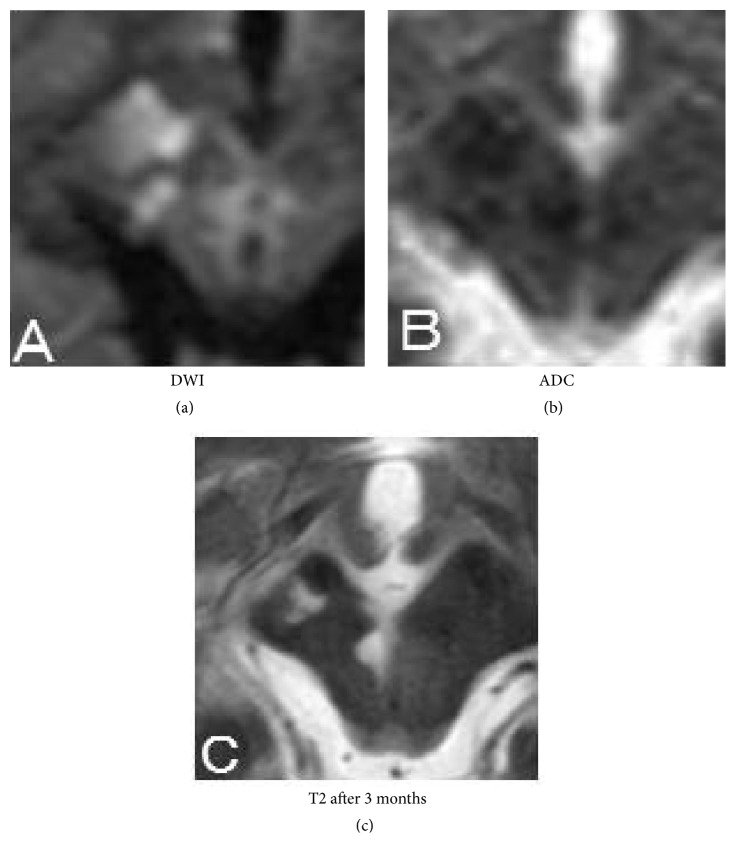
Head MRI on midbrain performed after aggravation of neurological symptoms. ADC map indicates the left paramedian lesion as stroke (a, b). Bilateral paramedian lesions three months after onset (c).
